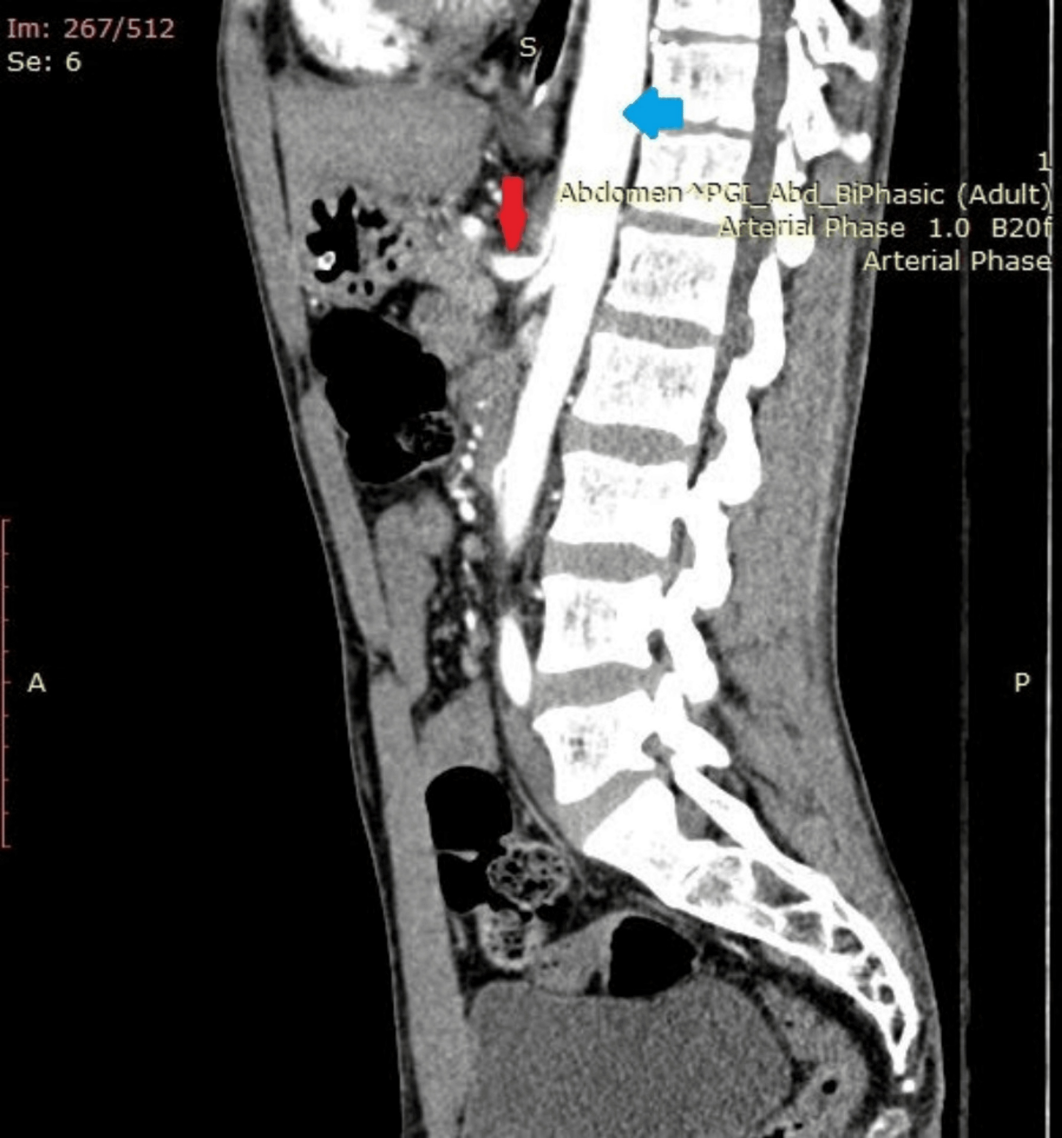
Preoperative contrast-enhanced CT scan of the abdomen (sagittal view) showing compressed celiac artery with post-stenotic dilation. Red arrow: celiac artery; Blue arrow: aorta; Yellow arrow: origin of superior mesenteric artery
